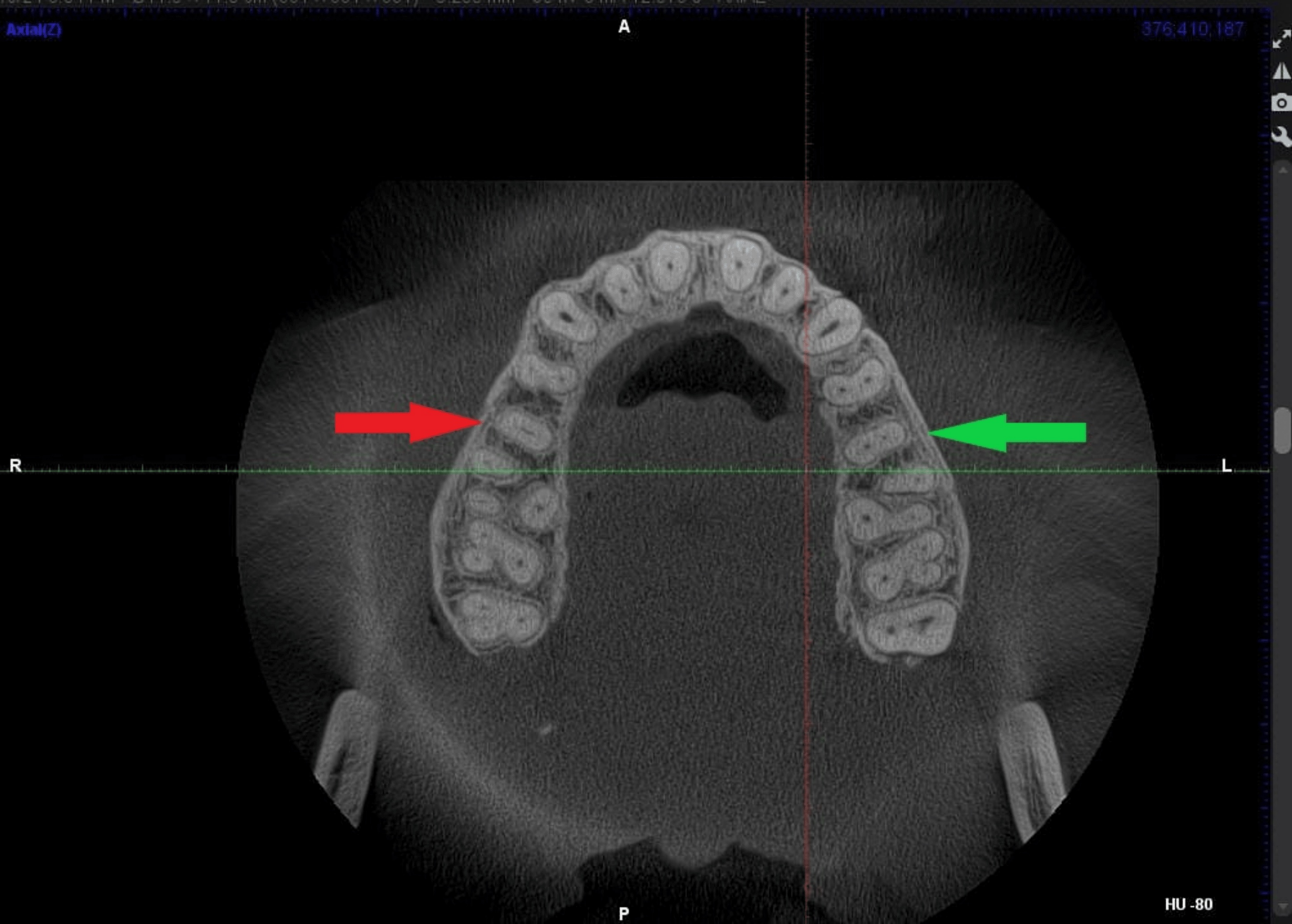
CBCT axial view showing Vertucci's Type I root canal morphology in the right upper maxillary second premolar (red arrow) and Vertucci's Type II root canal morphology in the left upper maxillary second premolar (green arrow) CBCT: cone-beam computed tomography
